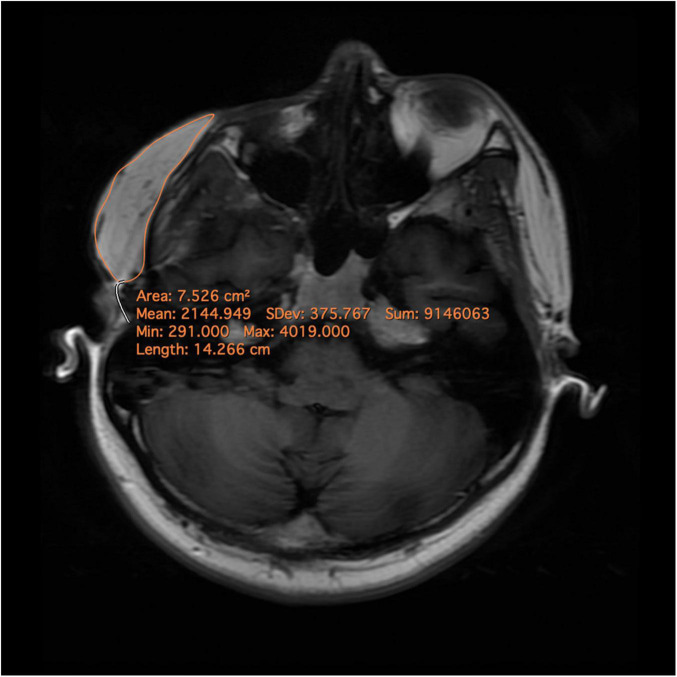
Magnetic resonance images and delineation of the subcutaneous adipose tissue. A region of interest (ROI) was precisely drawn on each axial slide by comprising pre- and post-operational scans. After ensuring that the entire targeted subcutaneous adipose tissue was included in the ROIs, the volumes were calculated automatically.
